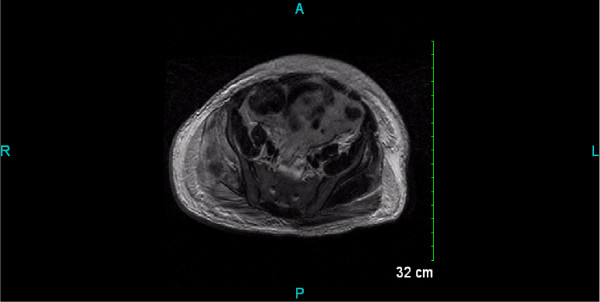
MRI pelvis. Enlargement of right gluteus minimus and medius muscles secondary to edema or hemorrhage with compartment syndrome.
